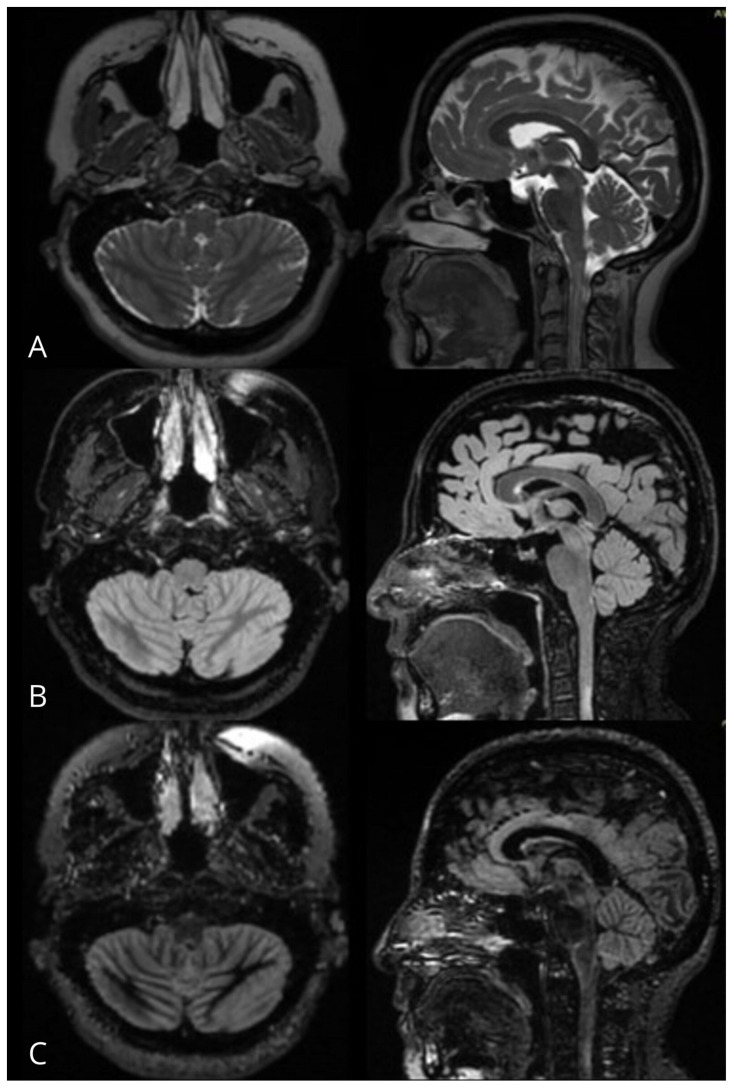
MRI of a patient with MS, in whom the area postrema cannot be identified in the 3D FSE Cube T2WI sequences (A) in axial and sagittal planes, but is visible in the axial and sagittal planes of 3D FSE Cube FLAIR (B) and 3D DIR (C) sequences.
